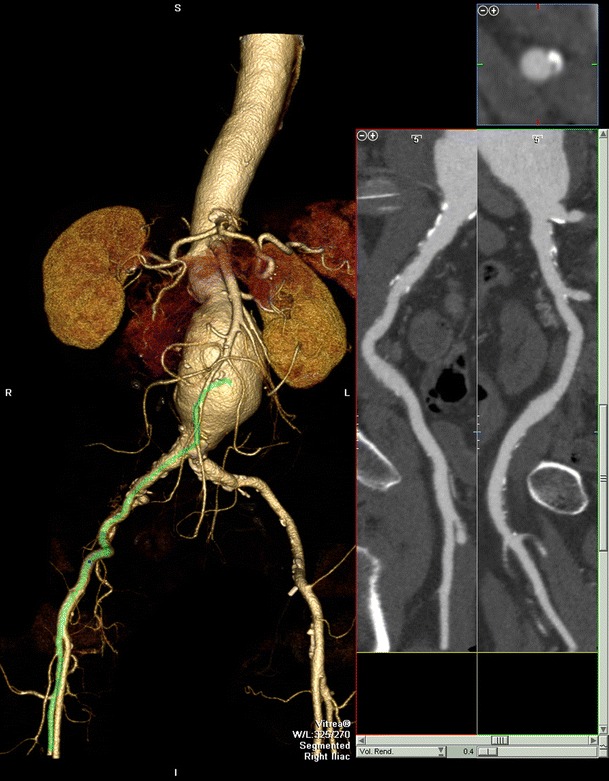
Three-dimensional volume-rendered image and curve planar reformat (CPR) along vessel centreline for right iliac arteries from CTA performed for assessment of abdominal aortic aneurysm to assess potential for endovascular repair. Infrarenal aneurysm terminates at the aortic bifurcation and CPR (delineated by green line on VR image) demonstrates satisfactory calibre right iliac access without stenotic disease
